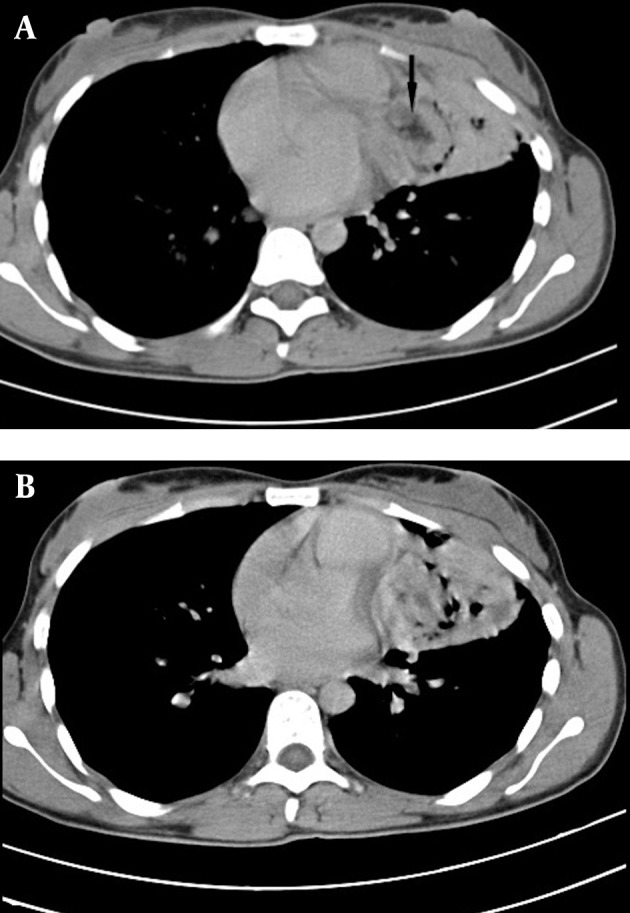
Figure 2. Contrast enhanced axial CT of thorax A, Heterogeneous mass containing fat (arrow), soft tissue, and gas, involving the mediastinum and lingular lobe B, Consolidation and ground-glass opacities seen in lingular lobe adjacent to the tumor
